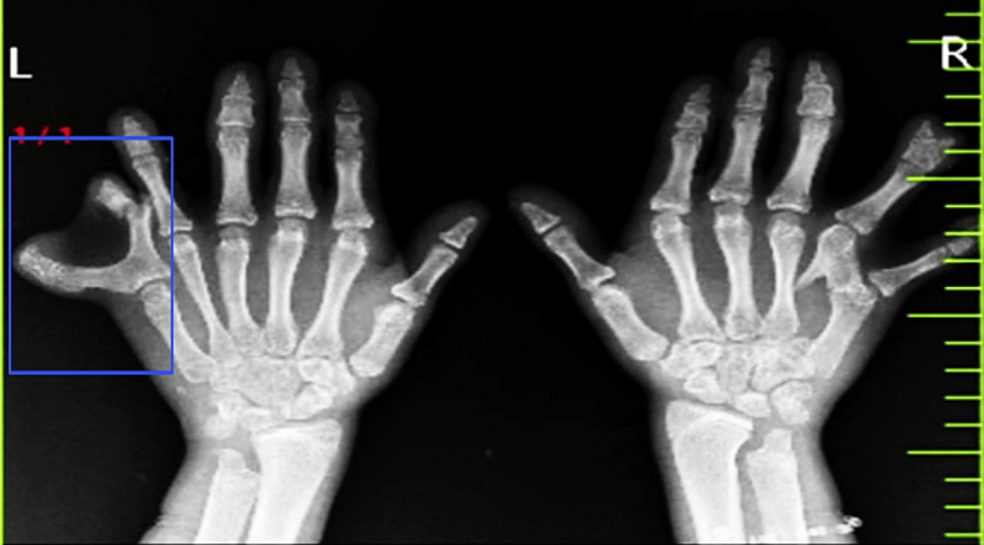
Radiography image of polydactyly in both hands Highlighted area marks polydactyly in the left hand
